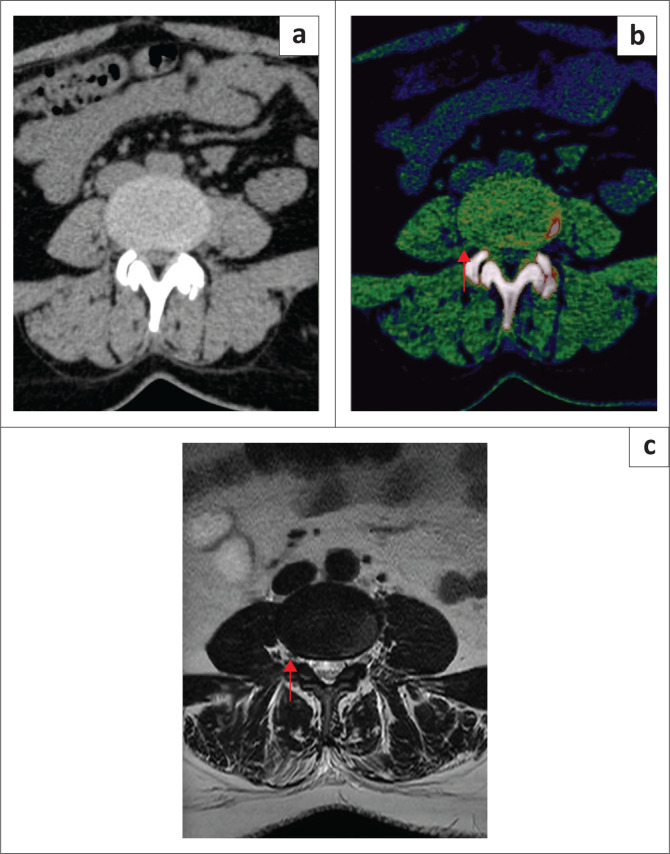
A 53-year-old woman who presented with lower back pain: (a) Bulging L4/L5 intervertebral disc with nerve root impingement of the exiting right L4 nerve root was underestimated on standard grayscale axial CT images. (b) Diagnosis of spinal nerve root impingement was made by all readers on the axial colour-coded electron-density reconstruction images. (c) Spinal nerve root impingement was confirmed on axial T2-weighted MRI (red arrow).
